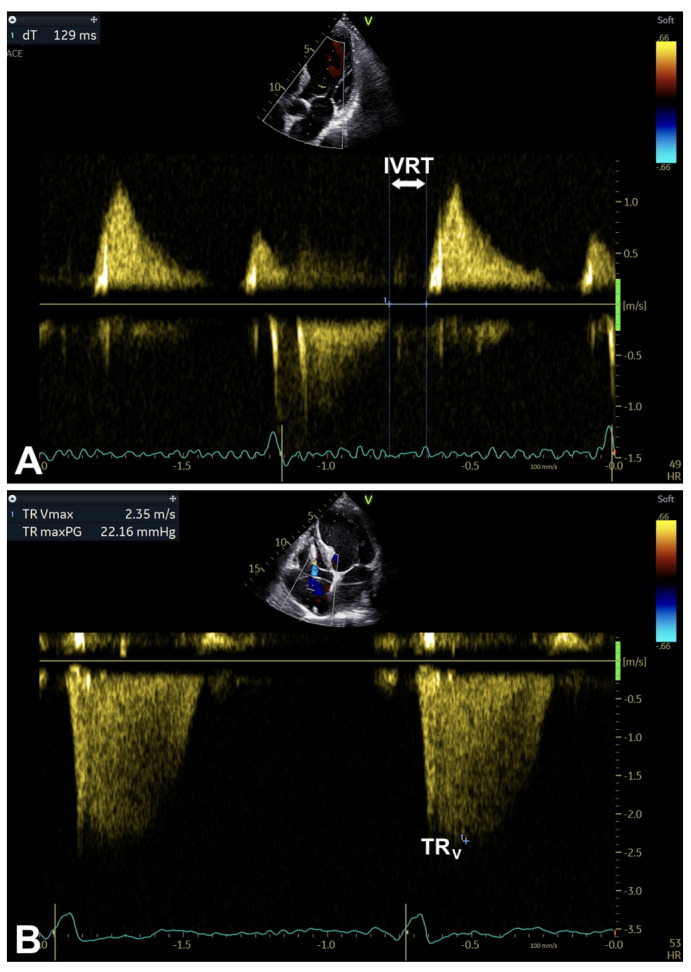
An example of IVRT evaluation (A) and CW Doppler study on TR velocity (B). These parameters may be helpful for contributing to assess diastolic function and LA pressure. CW, continuous-wave; IVRT, isovolumic relaxation time (measured in ms); TR, tricuspid regurgitation; TR_V_, peak TR velocity. Note: sweep speed at 100 mm/s (see text, tables, and references).
